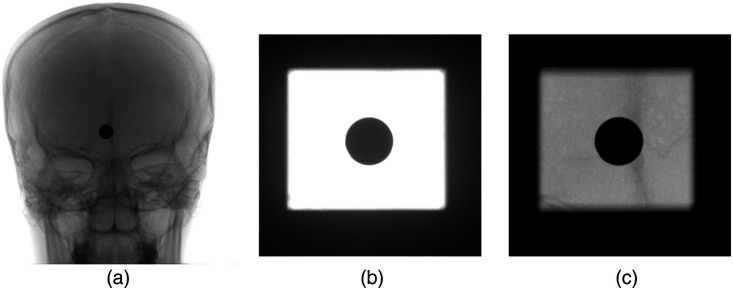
Resulting radiographs obtained by performing measurements with different settings of the X-ray angiography system. (a) Full head image acquisition with coil and without collimation. Measurement performed with collimation around coil but the system is (b) unaware of the presence of coil and (c) aware of the presence of coil. These acquisitions were performed to demonstrate reference point air kerma reduction and better visualization.
